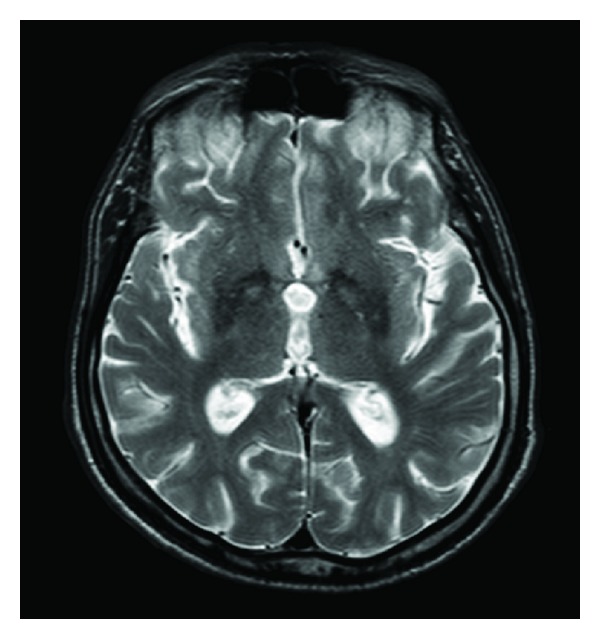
Axial T2 weighted MRI image showing “eye of the tiger” sign with bilateral hypointense rim of globus pallidus and area of central hyperintensity, in addition to hypointense posterolateral putamen.
